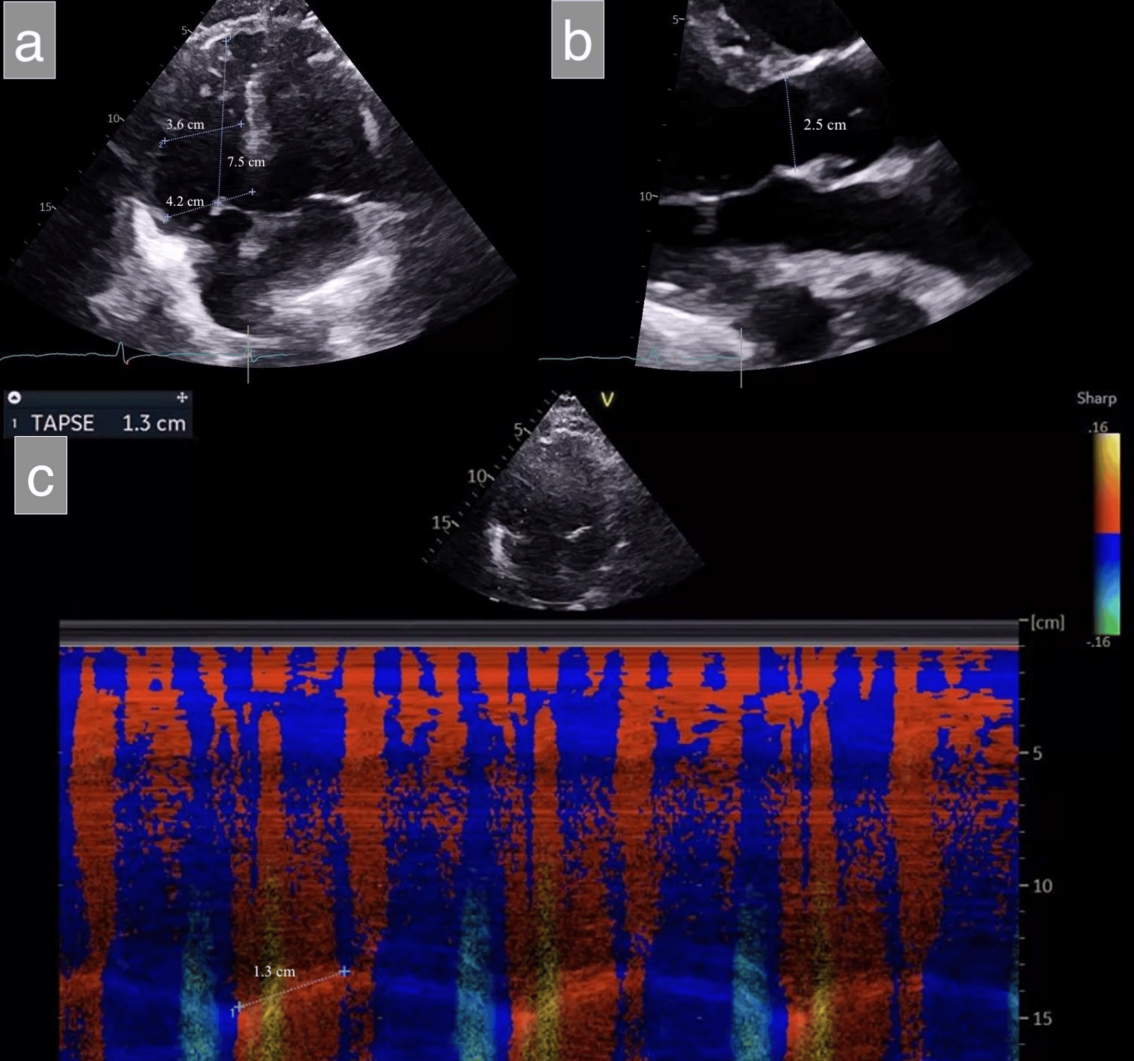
Transthoracic echocardiographic views demonstrating RV dilatation and impaired systolic function with preserved LV function (a) Apical four-chamber view showing a normal LV chamber with a slightly dilated RV. (b) Parasternal long-axis view demonstrating a normal LVOT (diameter 2.5 cm). (c) Color tissue Doppler echocardiogram showing reduced right ventricular systolic function with a TAPSE of 1.3 cm. LV, left ventricle; LVOT, left ventricular outflow tract; RV, right ventricle; TAPSE, tricuspid annular plane systolic excursion
